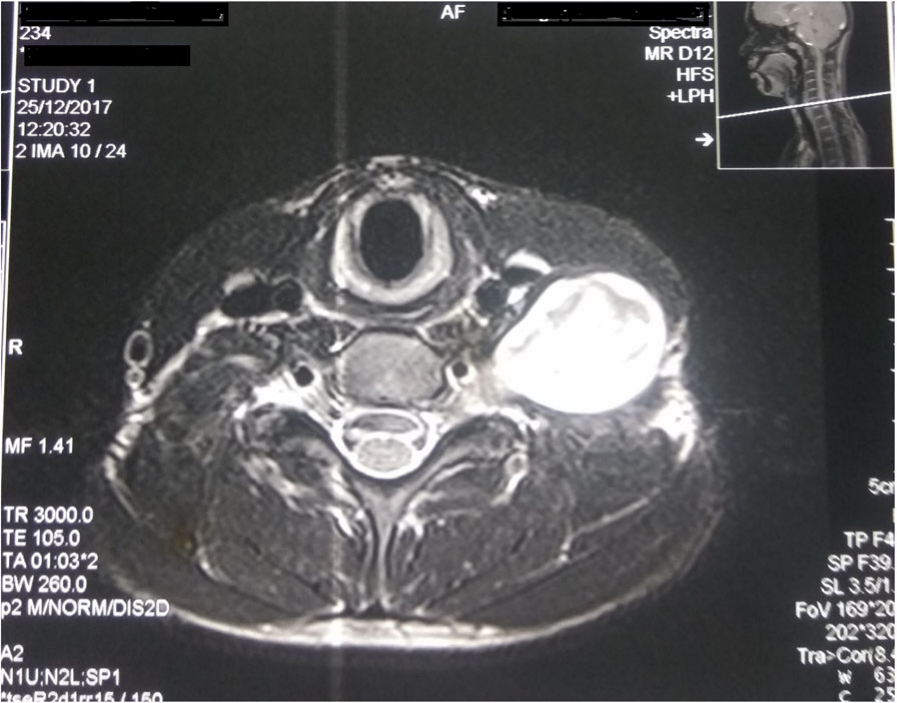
T2-weighted magnetic resonance imaging showing well-defined hyperintense mass in left posterior triangle extending up to neural foramina
